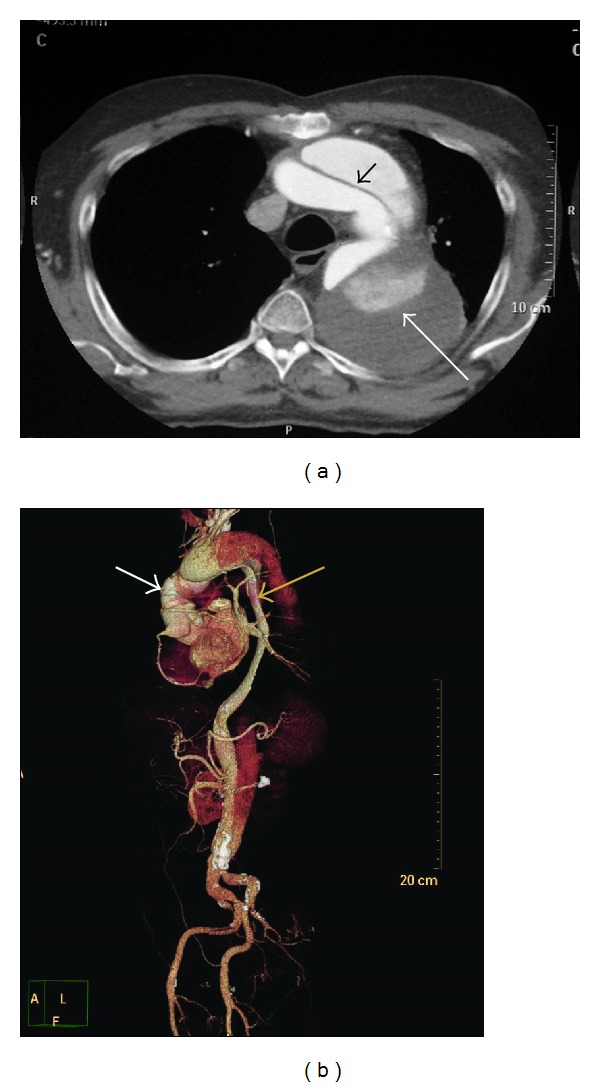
(a) Preoperative axial contrast-enhanced CT chest showing intimomedial flap in the aortic arch (black arrow) and partially thrombosed aneurysmal false lumen (white arrow). (b) CT angiogram, volume-rendered 3D image showing residual aortic arch and descending aortic dissection with aneurysmal degeneration (black arrow) following prior graft replacement of ascending aorta (white arrow) for type A aortic dissection.
